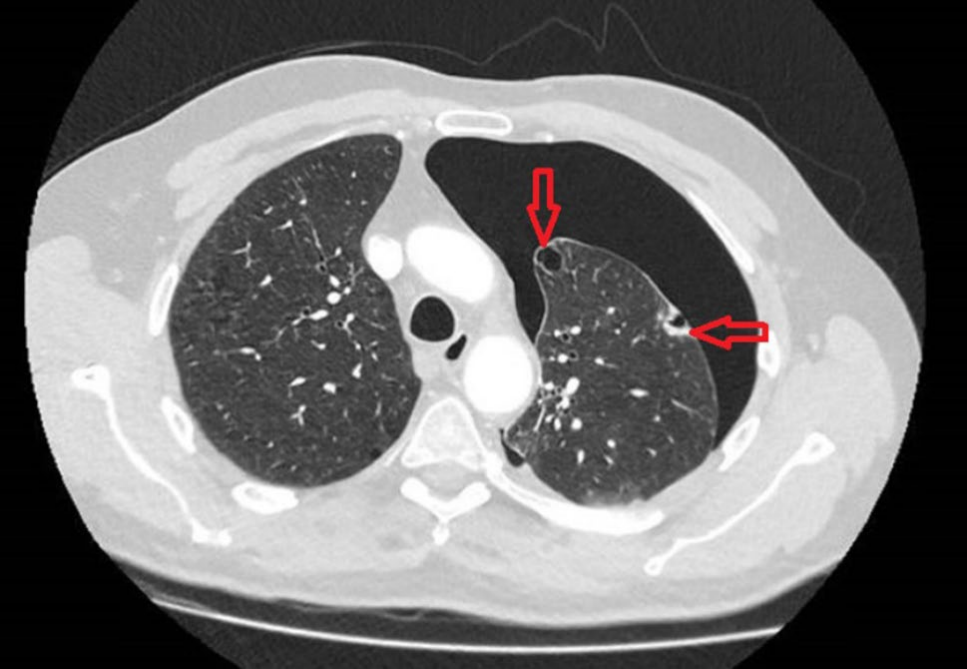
Shows an axial view of the CT taken on the patients second admission. Red arrows indicate significant bullous disease with the furthest right arrow showing the offending portion of the lung
